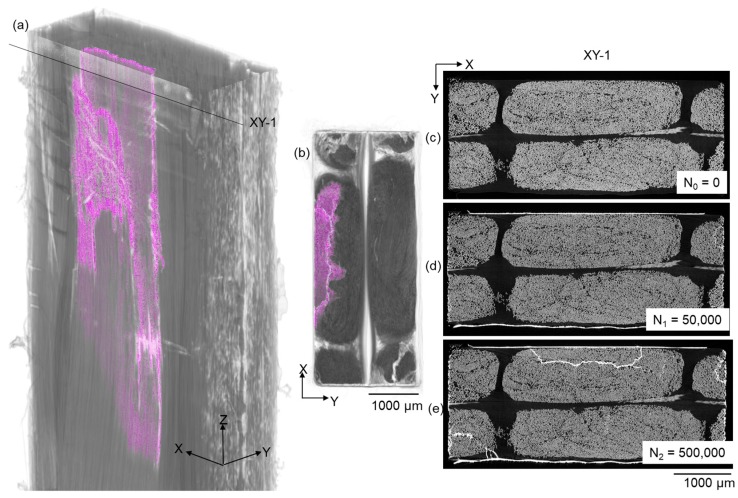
(a) Perspective and (b) plan view of the X-ray CT 3D volume rendering showing the extent of longitudinal splitting (purple), and an X-ray CT virtual XY section (position indicated in (a)) after (c) 0, (d) 50,000 and (e) 500,000 cycles showing the evolution of longitudinal splits.
